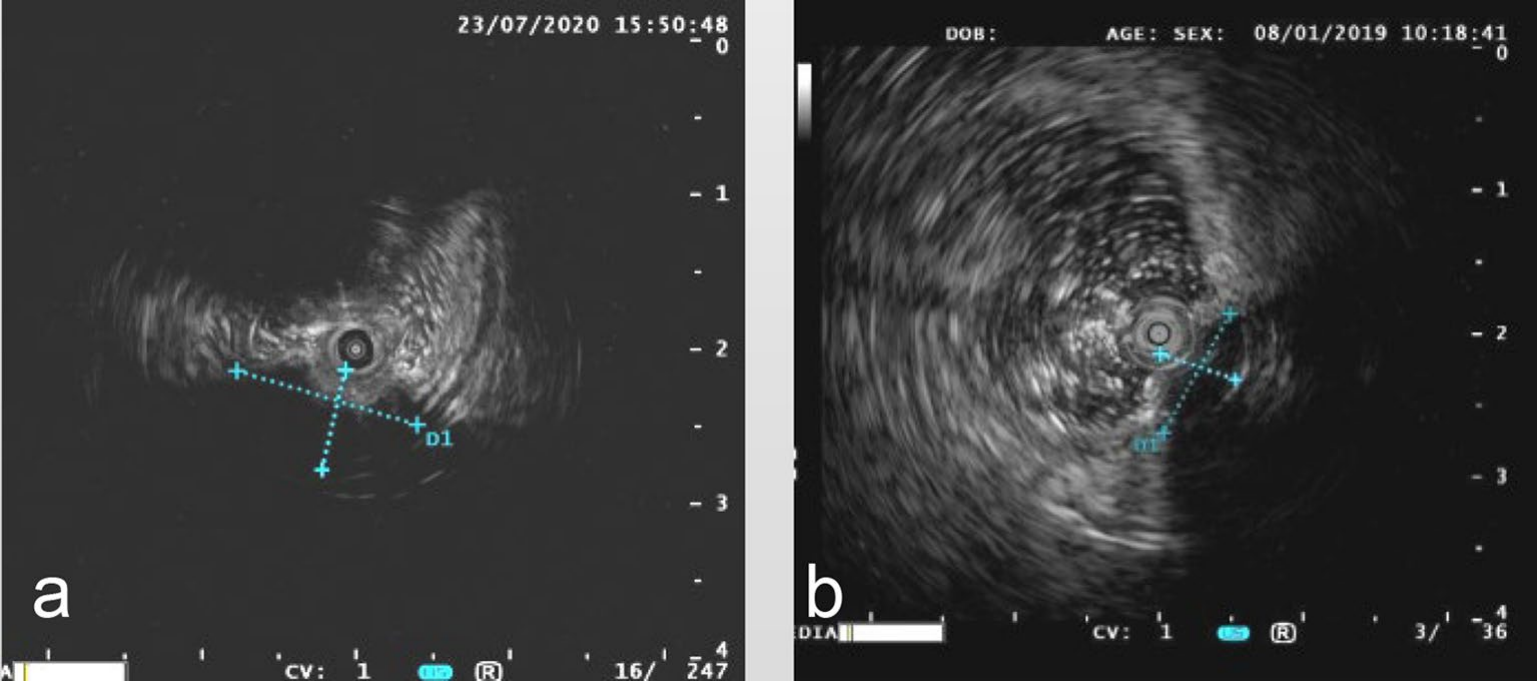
Colonoscopic ultrasound. (a) A hypoechoic mass is observed in the submucosa, which was demarcated from the muscularis propria. (b) A hypoechoic mass in the submucosa that is poorly demarcated from the muscularis propria.
